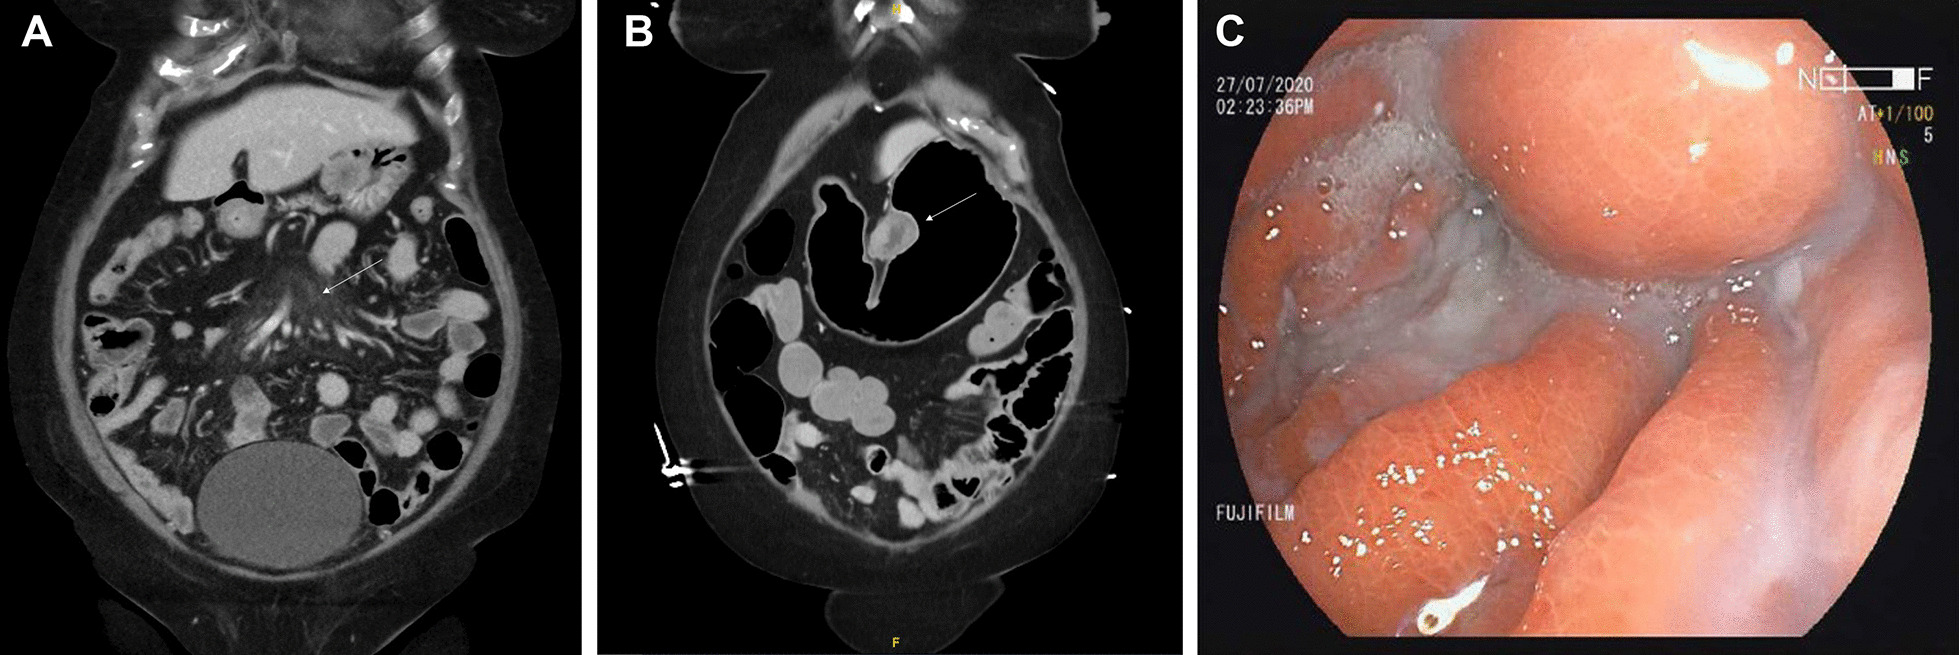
A Abdominal computed tomography (CT) on admission revealed misty stranding of the mesentery with small-volume mesenteric nodes suggestive of mesenteric panniculitis. B Abdominal CT 20 days later uncovered an intramural mass along the lesser curvature of the stomach. C Upper gastrointestinal endoscopy found a gastrointestinal stromal tumor (GIST) along the lesser curve of the stomach
